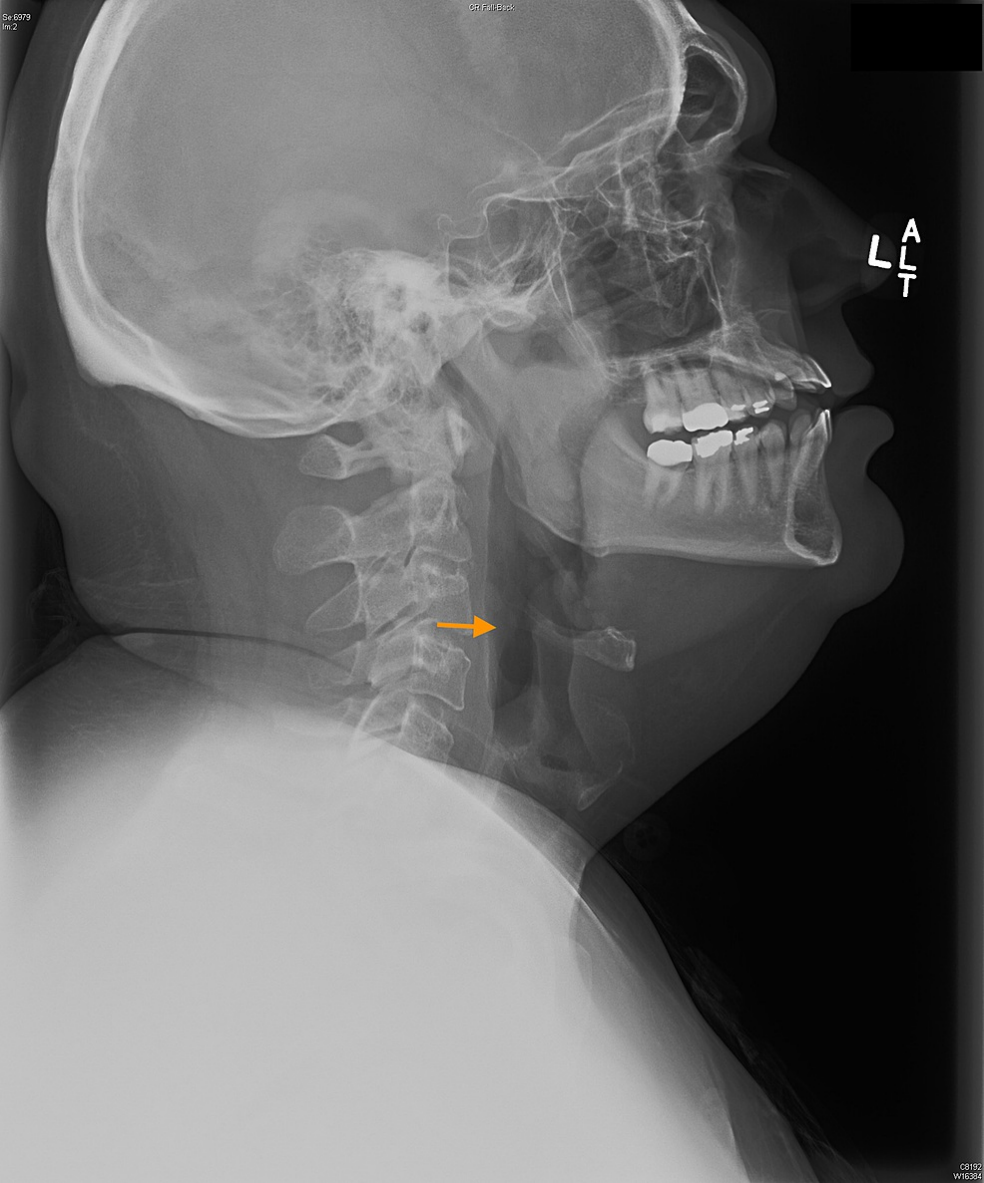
Lateral view radiograph of soft tissue of neck 2 hours and 37 minutes later demonstrating much-improved airway (arrow)
